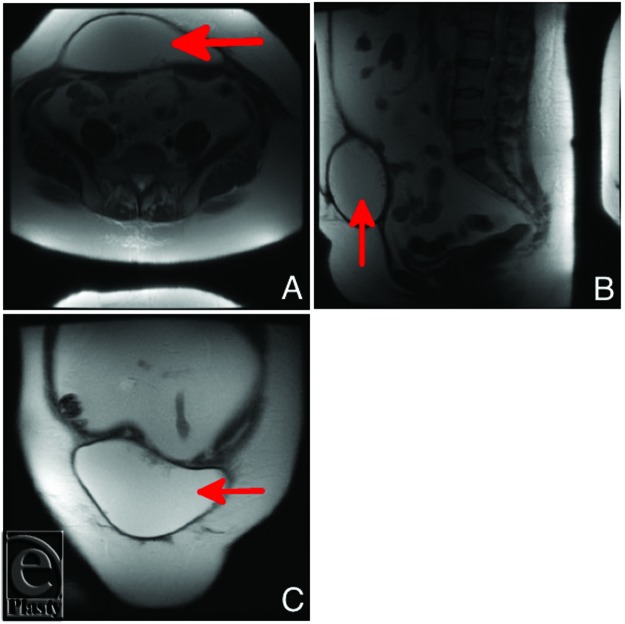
MRI scan of a 50-year-old patient done 21 months after immediate breast reconstruction. The patient had previously undergone excision of a seroma sac (“capsulectomy”) 12 months prior to the imaging. The scan shows a high signal intensity, nonloculated lesion on T2W measuring approximately 19 × 7 × 11 cm^3^ in the subcutaneous tissues immediately anterior to the rectus muscles. (*a*) Transverse view, (*b*) Sagittal view, and (*c*) Recumbent view showing the size of the seroma and its location superficial to the abdominal musculature. MRI indicates magnetic resonance imaging.
